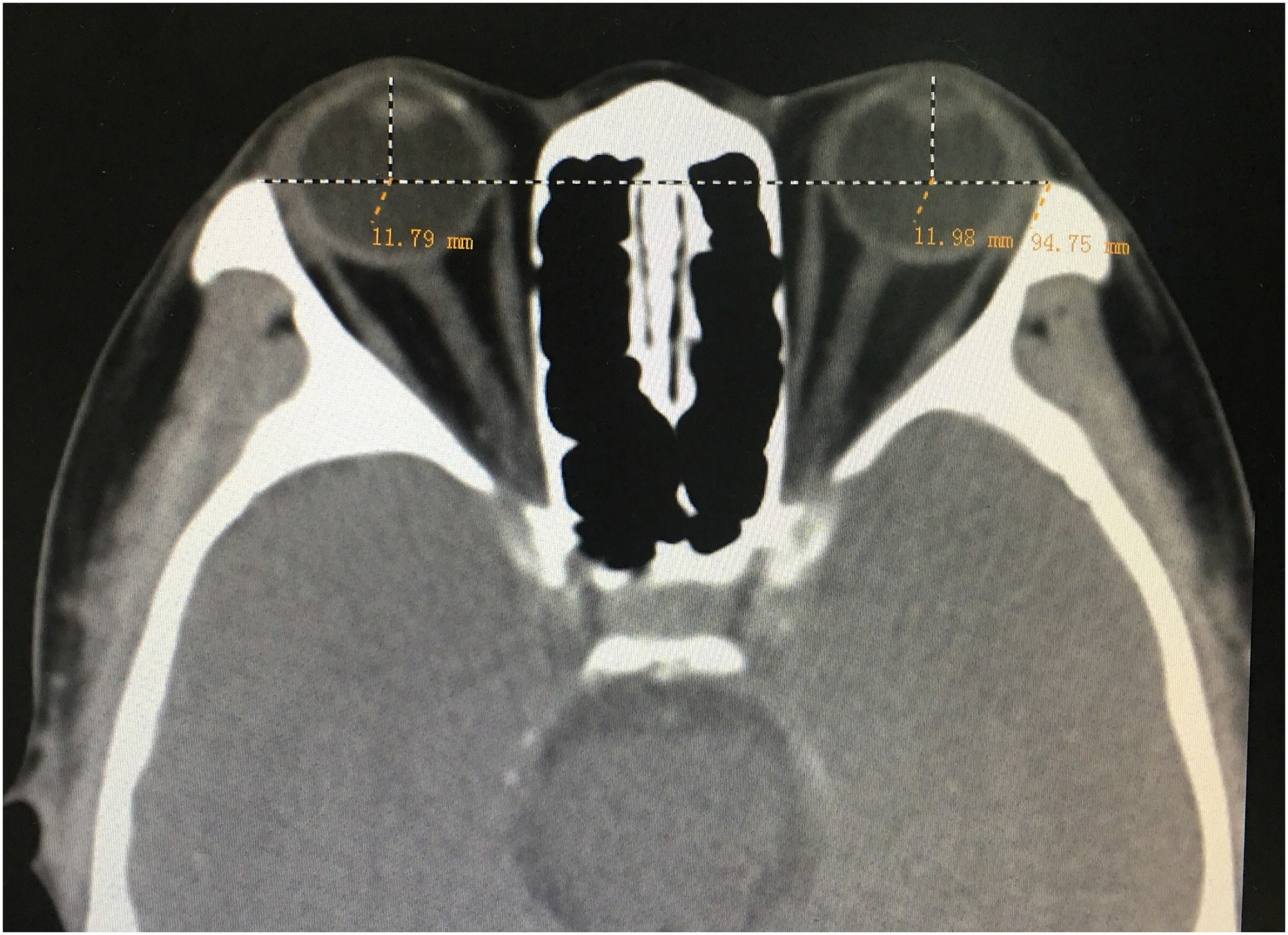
It was the transverse section of head scanning by computed tomography. An interzygomatic line was drawn on the axial view image when the lens was seen clearly. A perpendicular line was drawn from the inner corneal surface to the interzygomatic line, bisecting the lens.
